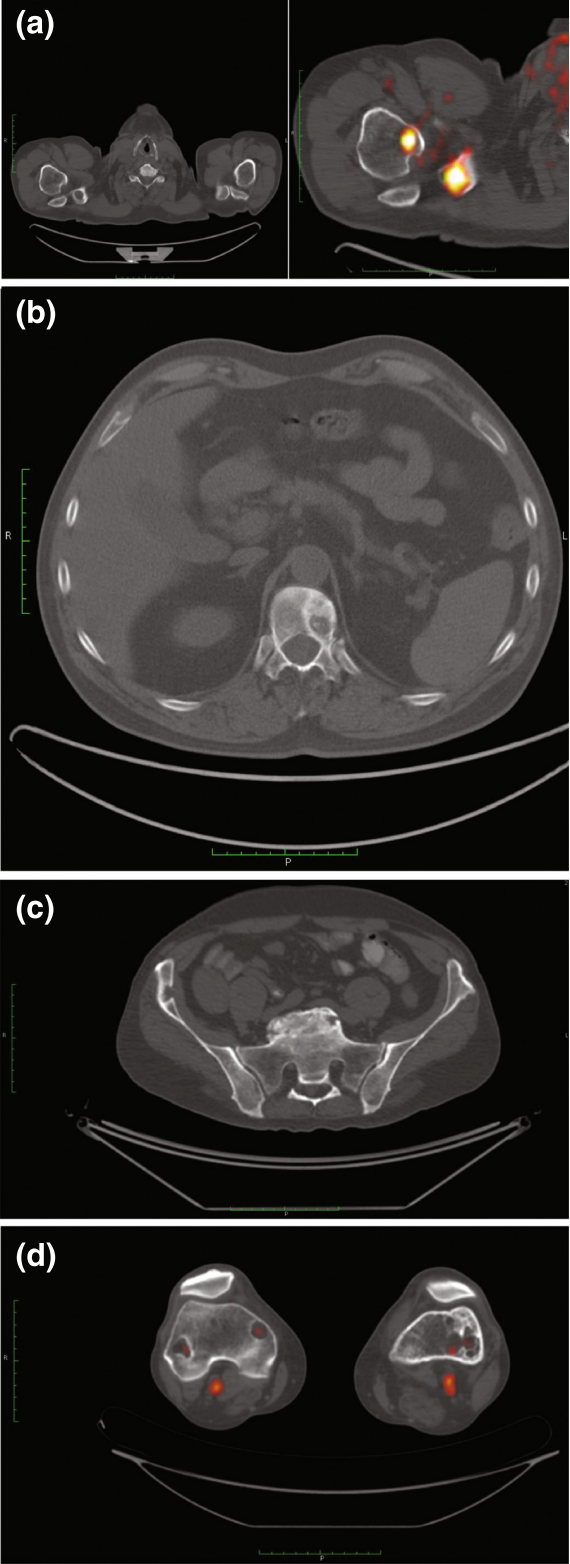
Positron emission tomography-computed tomography imaging of bone lesions. (a) Acromial extremity of the right clavicle, March of year 4. (b) Thoracic vertebra T12, March of year 4. (c) Right ilium, March of year 5. (d) Distal extremity of the femur bones, March of year 5.
